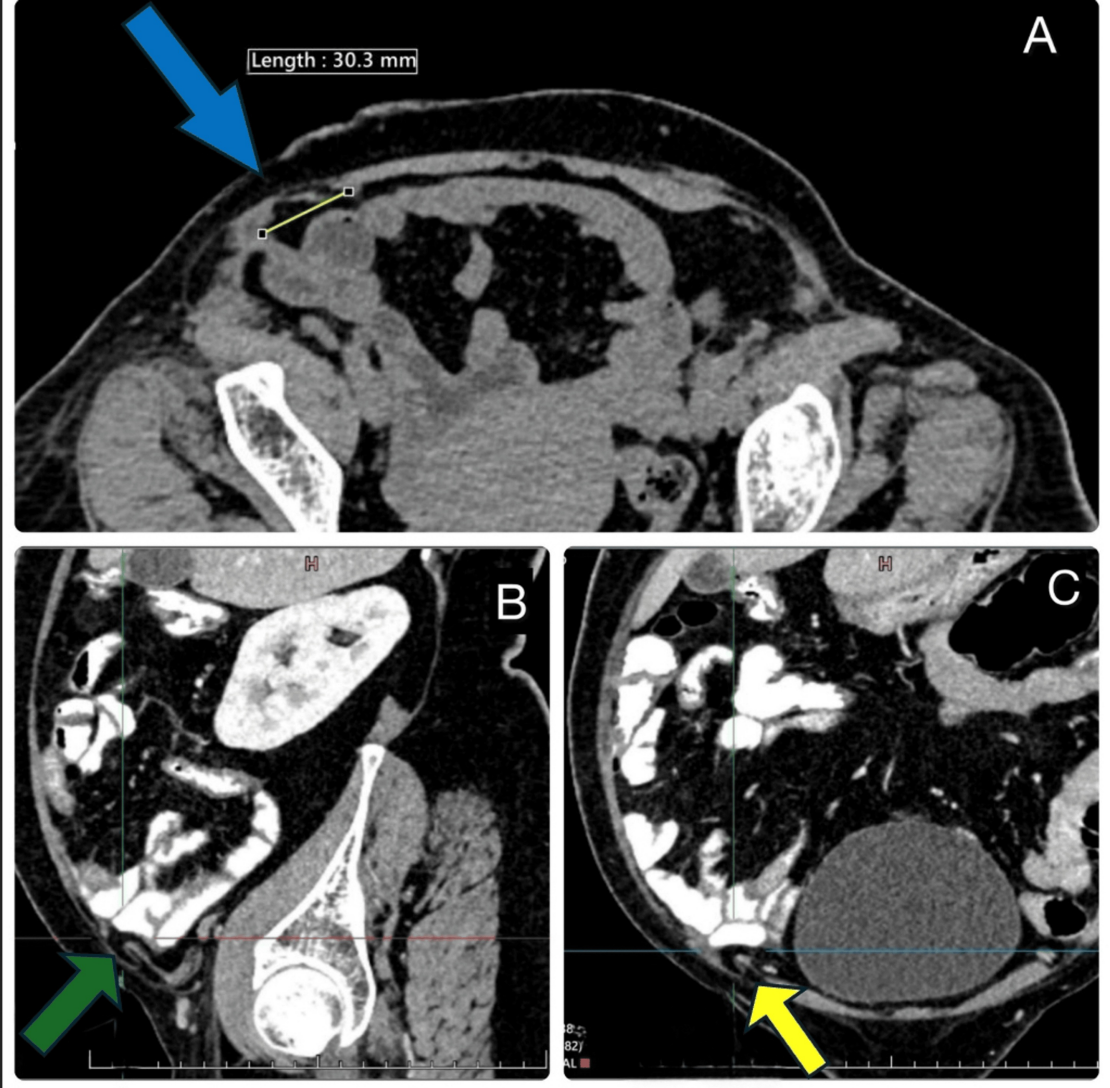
CECT abdomen revealed a defect in the Spigelian fascia on the right side, with herniation of bowel loops consistent with a Spigelian hernia (A) Axial CECT of the abdomen revealed a defect in the Spigelian fascia on the right side (blue arrow), with herniation of bowel loops consistent with a Spigelian hernia. There was no evidence of bowel obstruction, incarceration, or strangulation, (B) sagittal CECT abdomen shows a defect as mentioned above (green arrow), and (C) coronal CECT abdomen shows a defect as mentioned above (yellow arrow). CECT: contrast-enhanced computed tomography
